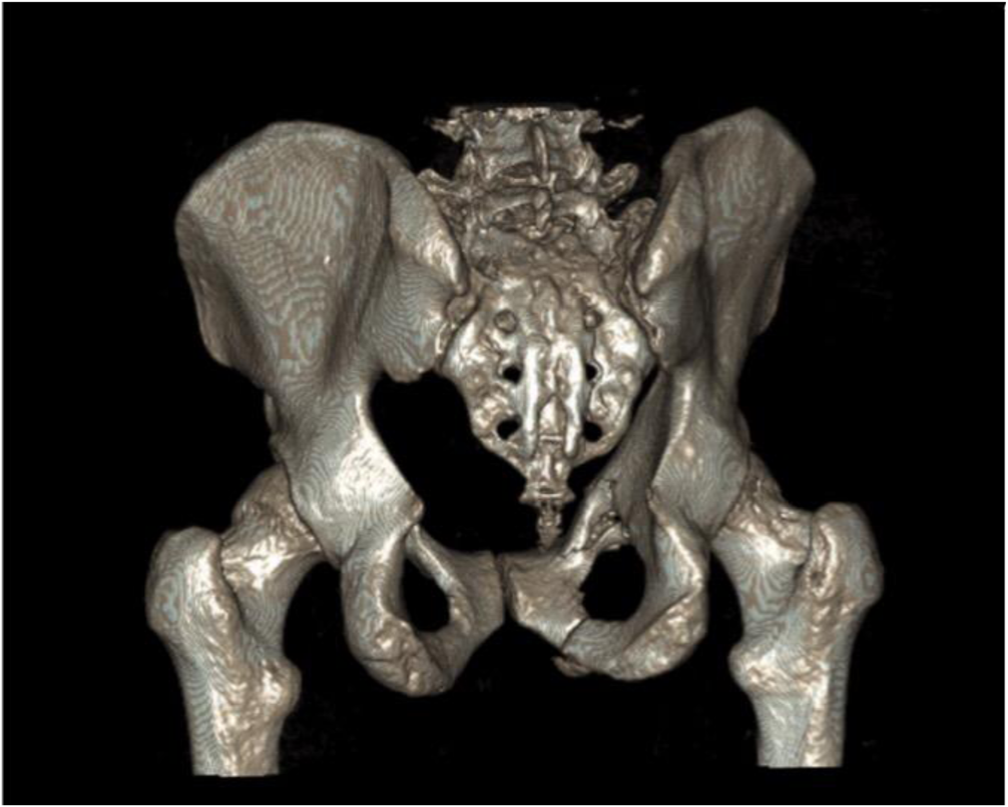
CT 3D reconstruction of Figure 5 injury.
